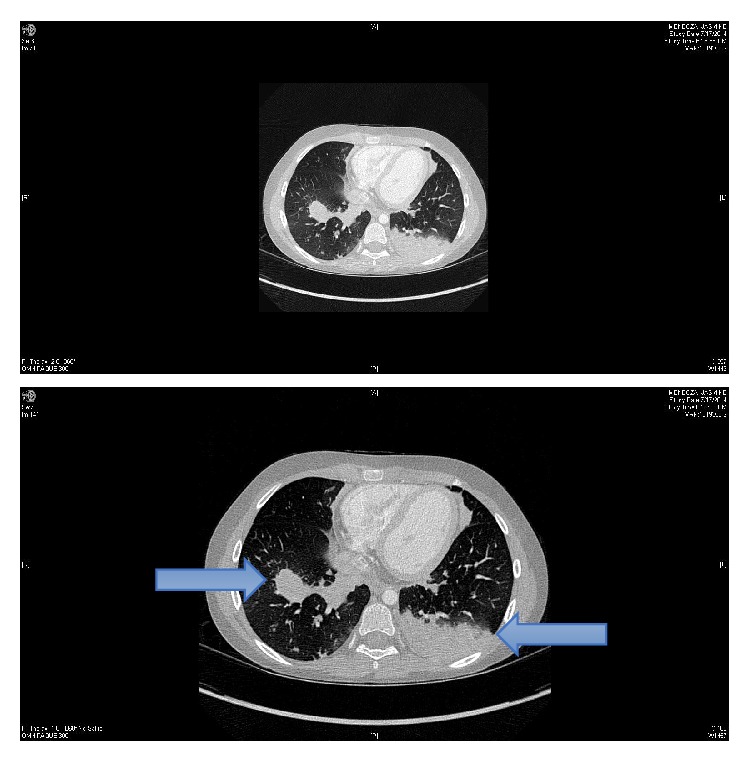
Chest CT, axial view with multifocal pneumonia.
